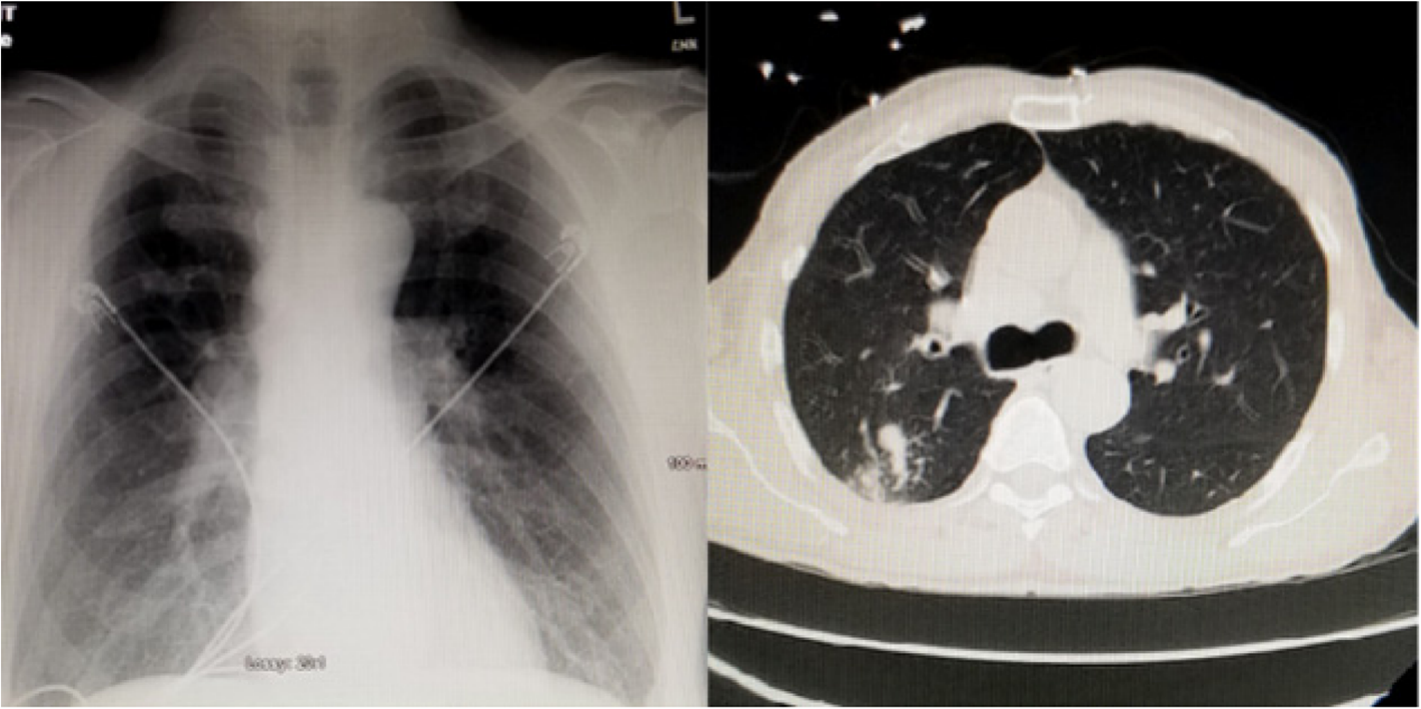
Left: AP chest plain film read as no acute cardiopulmonary disease. Right: CT chest showing a conglomeration of nodules and opacities measure 3.0 × 1.9 cm of the inferior portion of the right upper lobe
